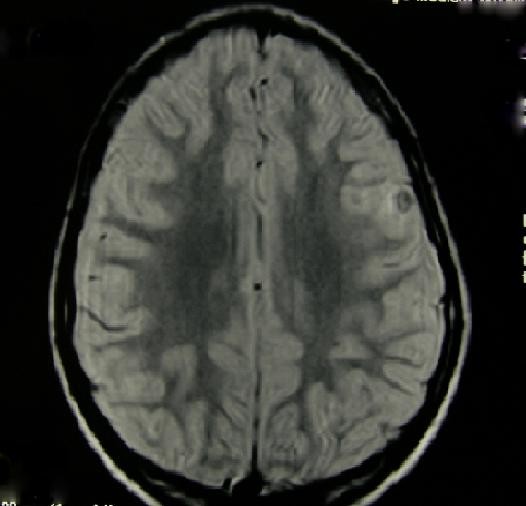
Post treatment brain MRI. Axial T2-weighted MRI shows edema's disappearance and calcified lesions, one in right lentiform nucleus and one in left frontal-parietal cortex.
